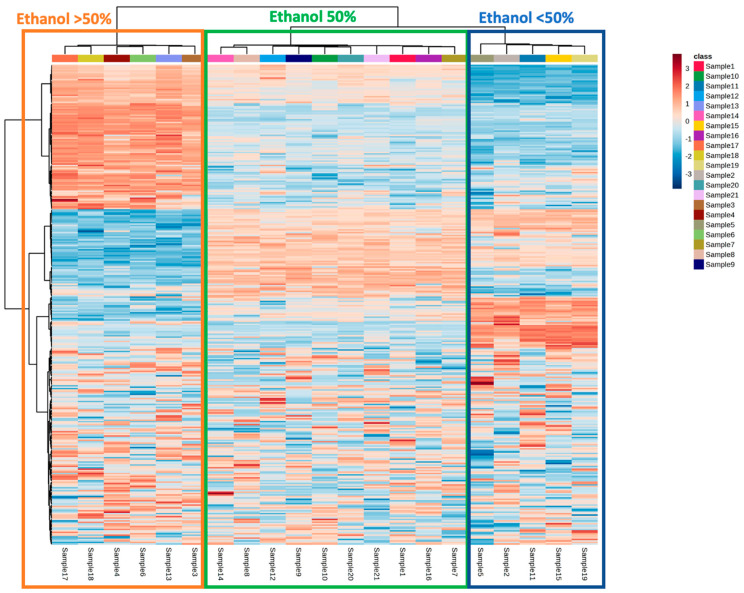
Unsupervised hierarchical cluster analysis (HCA) carried out considering the phytochemical profile of the different 21 duckweed extracts as a function of extraction temperature, ultrasound power, and % of ethanol.
